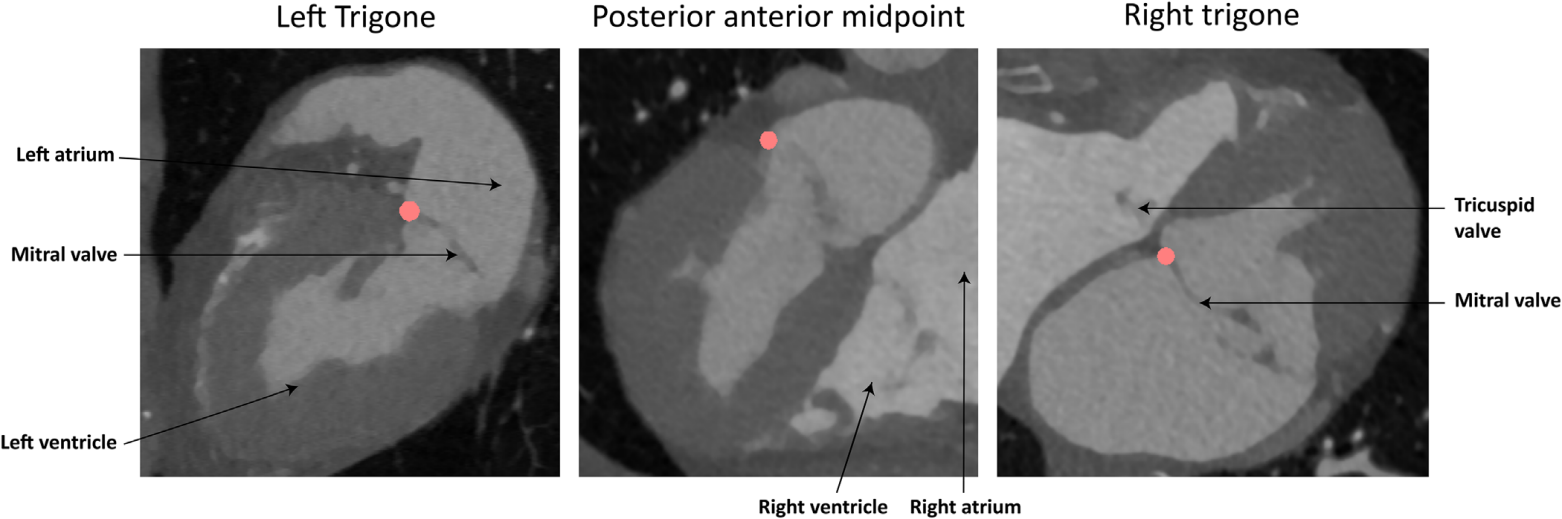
The CT slices display the location of the left trigone, posterior–anterior midpoint, and right trigone of the mitral valve.
